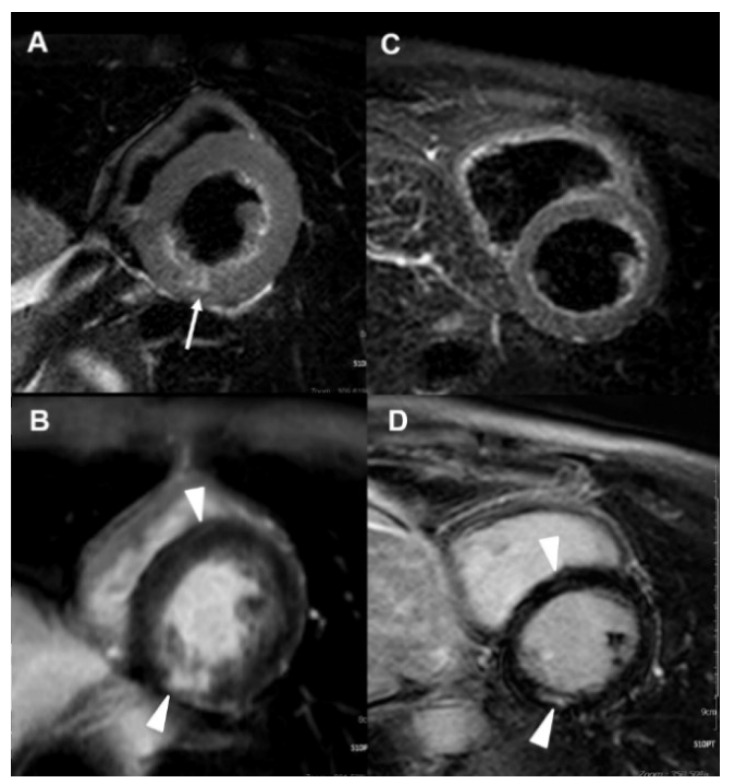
Serial cMRI images in case no. 2 before and after RTX therapy. (A,B) Pre-RTX treatment cMRI images revealed patchy edema in short-axis T2-weighted images (A, white arrows) and post-gadolinium delayed enhancement images (B, white arrowheads). (C,D) After induction and two courses of maintenance therapy, resolved myocardial edema (C) and some mid-wall fibrosis at the sepal and inferior walls of LV mid-cavity (D, white arrowheads).
